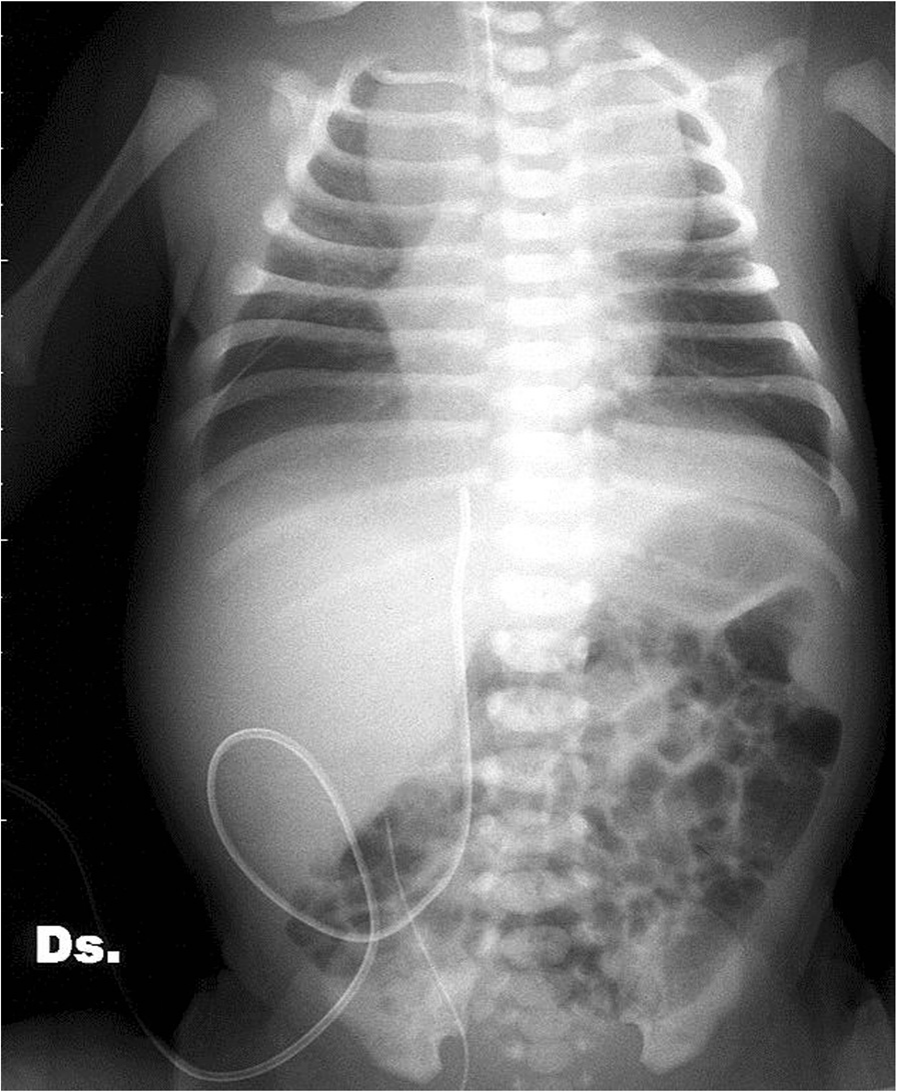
Chest X-ray on day 3: pleural effusion resolution; relevant mediastinum enlargement
